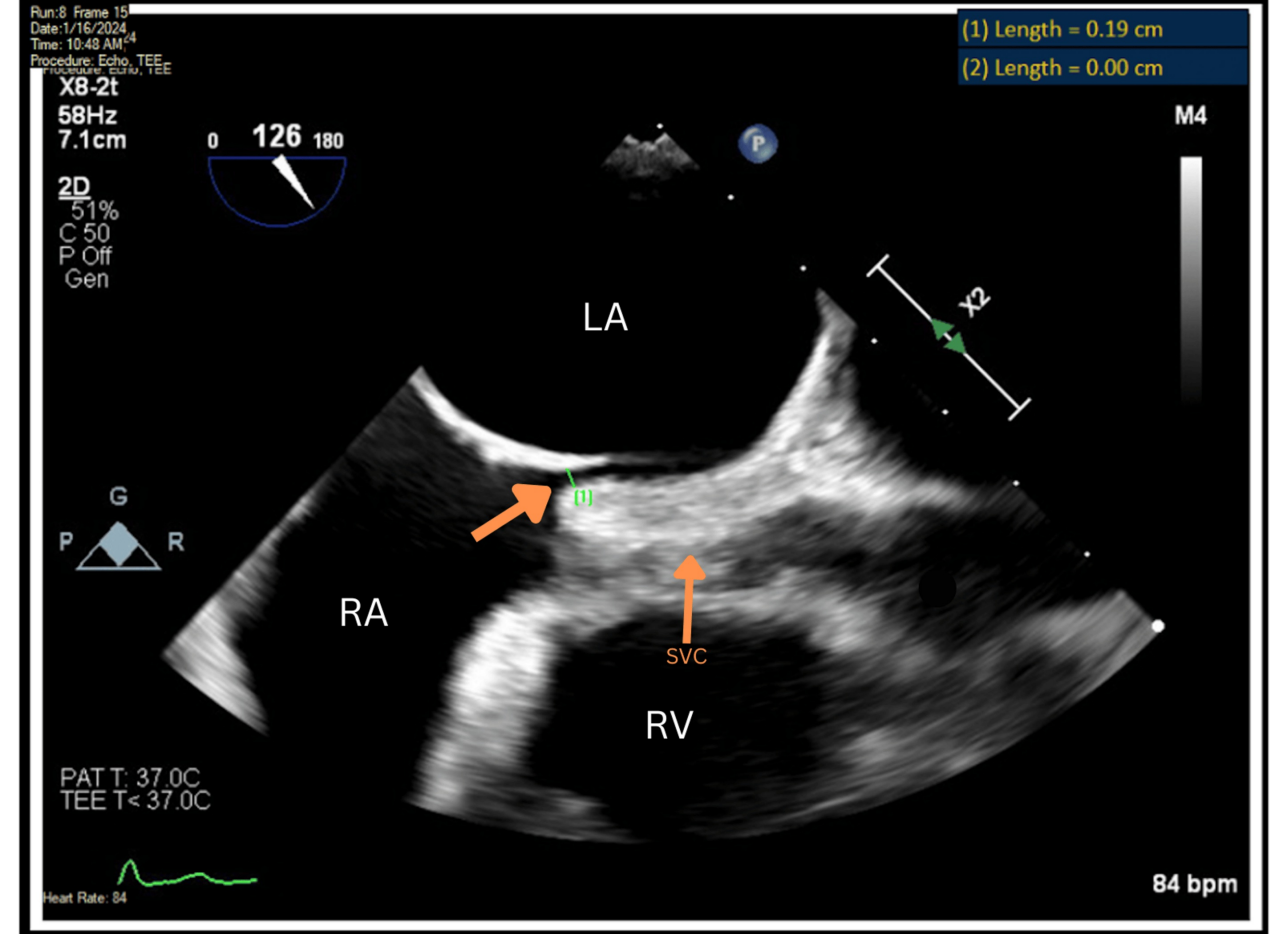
Transesophageal echocardiogram demonstrating a primum ASD. The left orange arrow highlights the measured defect (0.19 cm) between the right atrium (RA) and left atrium (LA). The right orange arrow identifies the superior vena cava (SVC). Cardiac chambers are labeled: RA = right atrium; LA = left atrium; RV = right ventricle. ASD: atrial septal defect.
